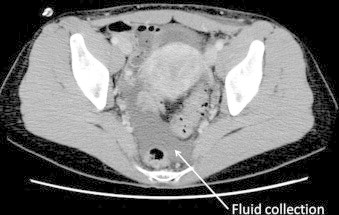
A contrast-enhanced CT scan shows a little ascites at pelvic space (white arrow).
